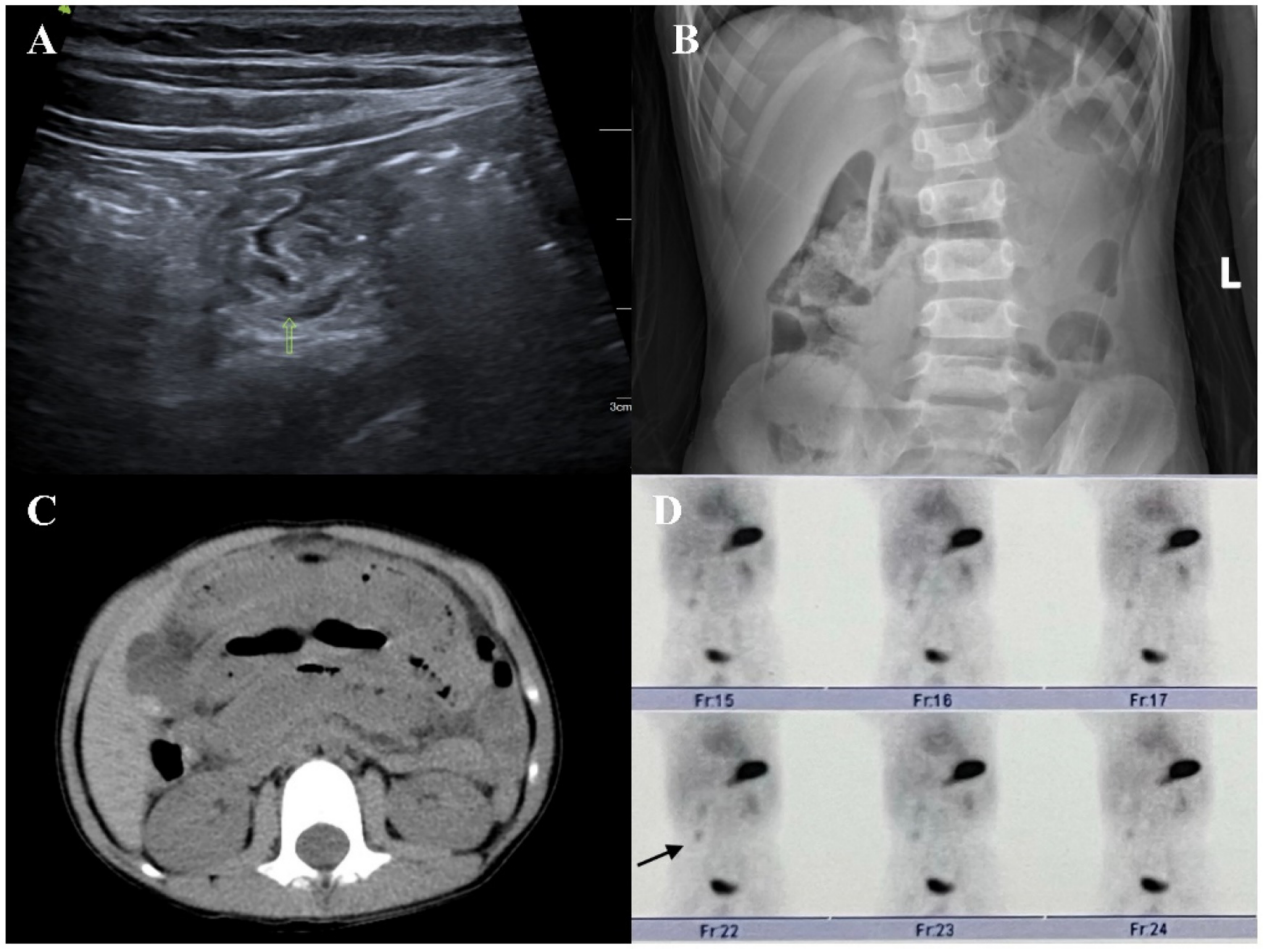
(A) On an abdominal ultrasound image, an abnormal intestinal tract can be seen in the right abdomen, where one end is connected with the intestinal tract and the other end is a blind end. A small amount of liquid dark area can be seen on the wall. (B) Abdominal plain film image showing a small amount of liquid and a plane of air in the abdominal intestinal tract. (C) Abdominal computed tomography image showing part of the intestinal dilatation and effusion, edema, and thickening of the intestinal wall. (D)
